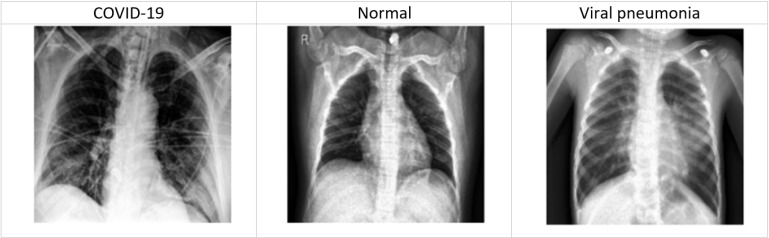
Example of chest X-ray images.
